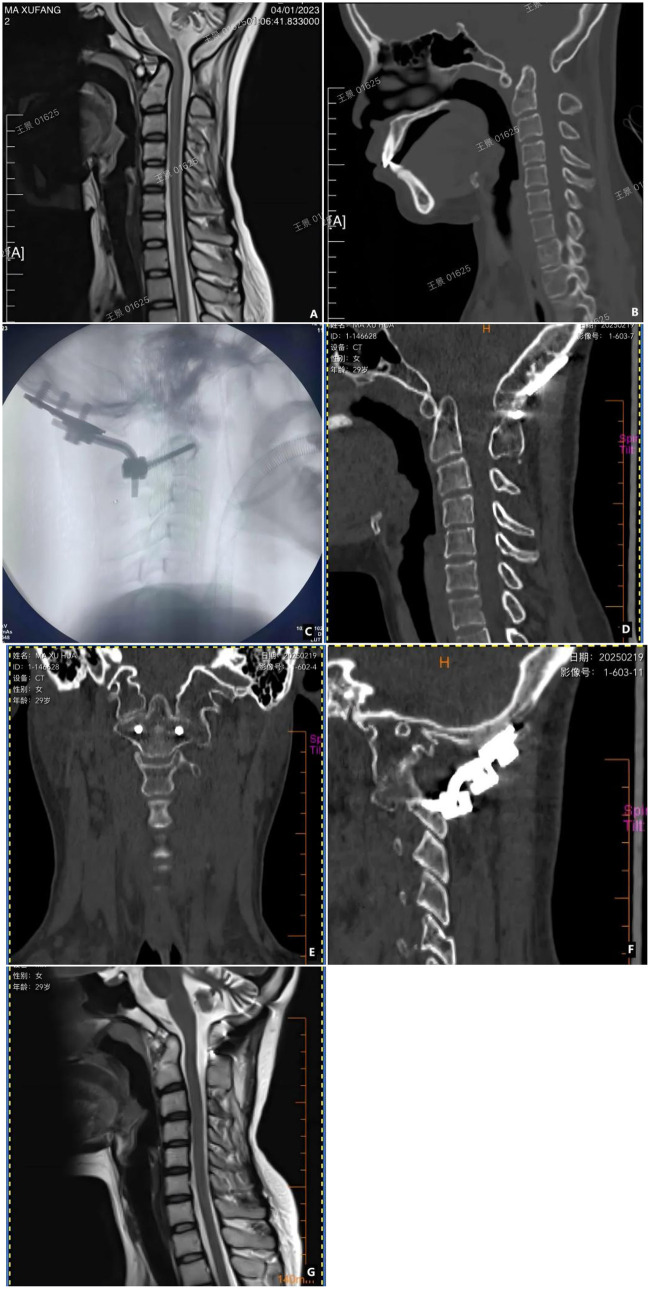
Representative case. The female patient presented with gait instability and a “tongue-shortening” sensation with dysarthria triggered by prolonged speaking for 6 months, and was diagnosed with AAD, NTS, and congenital occipito-cervical fusion. She underwent posterior reduction and occipito-cervical fusion. Gait instability improved postoperatively, and NTS symptoms completely resolved at 27-month follow-up. (A) Preoperative sagittal T2-weighted MRI showing separation at the atlanto-odontoid complex with posteriorly displaced odontoid compressing the spinal cord. (B) Preoperative sagittal 3D-CT reconstruction demonstrating atlantoaxial dislocation. (C) Intraoperative fluoroscopy during posterior reduction and occipito-cervical fusion. (D) Sagittal 3D-CT at 27 months showing satisfactory reduction of the atlantoaxial joint. (E) Coronal CT demonstrating osseous fusion across the LAAJ. (F) Sagittal 3D-CT showing interfacet fusion. (G) Follow-up MRI confirming reduction of the atlanto-odontoid complex and decompression of the spinal cord.
